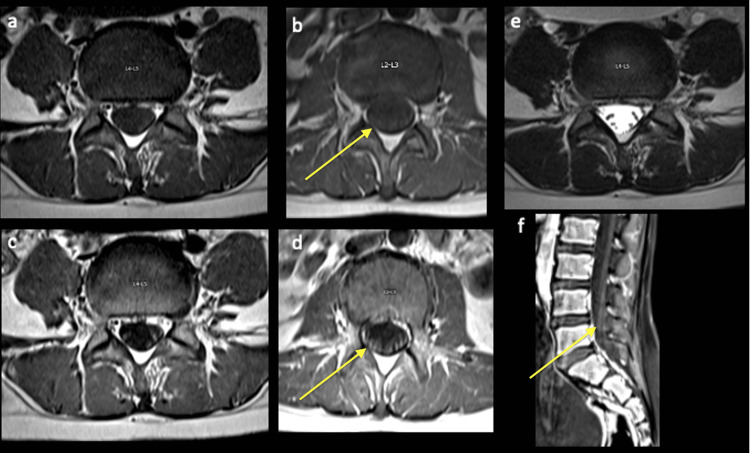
Axial T1-weighted (a- L4-L5, b L2-L3), gadolinium-enhanced T1-weighted (c- L4-L5, d L2-L3), axial T2-weighted (e, L4-L5), and sagittal gadolinium-enhanced T1-weighted (f) images demonstrating mild diffuse thickening and enhancement of cauda equina (enhancement marked by yellow arrows).
